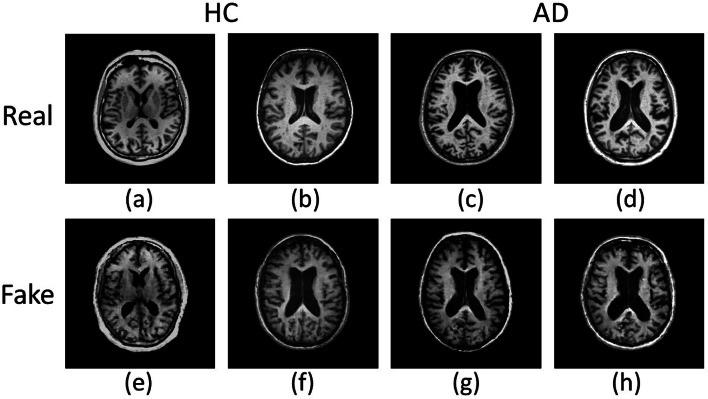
Comparison of synthetic images generated by the PACGAN with real brain MRI. (a, b) display real brain MRI of healthy controls (HC), while (c, d) depict real brain MRI images of Alzheimer’s Disease (AD) subjects. Image Data IDs from the ADNI dataset: I217939, I1391580, I50702, I1190066, respectively. On the lower row, (e, f) present synthetic brain MRI images of HC subjects, while (g, h) show synthetic brain MRI images of AD subjects.
